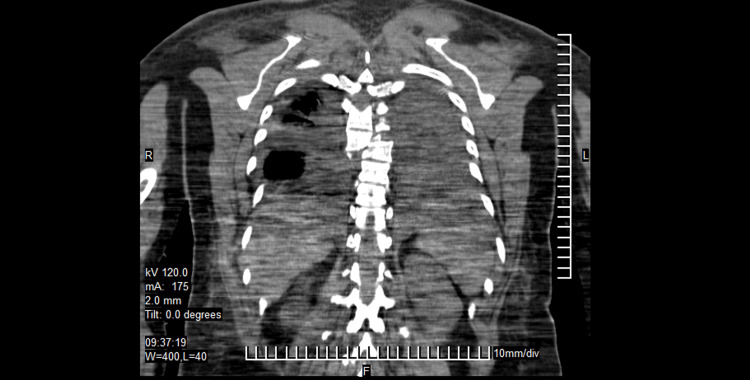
Case 1 CT scan coronal cut showing the lateral displacement of the vertebral bodies to the right and bilateral hemothorax, predominantly on the left side.
